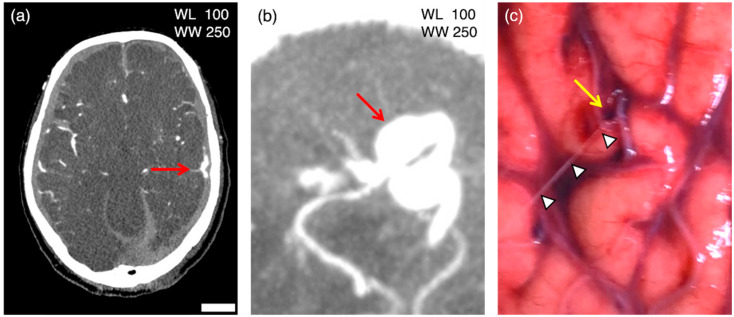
Postmortem computed tomography angiography (PMCTA) images (a, axial image; b, multi-planar reconstruction image) and macroscopic image of the brain in case 6. PBS perfusion in the left internal carotid artery after craniotomy led to PBS spurting (arrowheads) from the ruptured cortical artery with marginal bleeding (yellow arrow) on the left parietal lobe. PMCTA images show extravascular leakage of the contrast agent (red arrows) at a site consistent with cortical arterial rupture. On the lower right side of (a), the bar is equal to 25 mm for (a) and 5 mm for (b,c).
